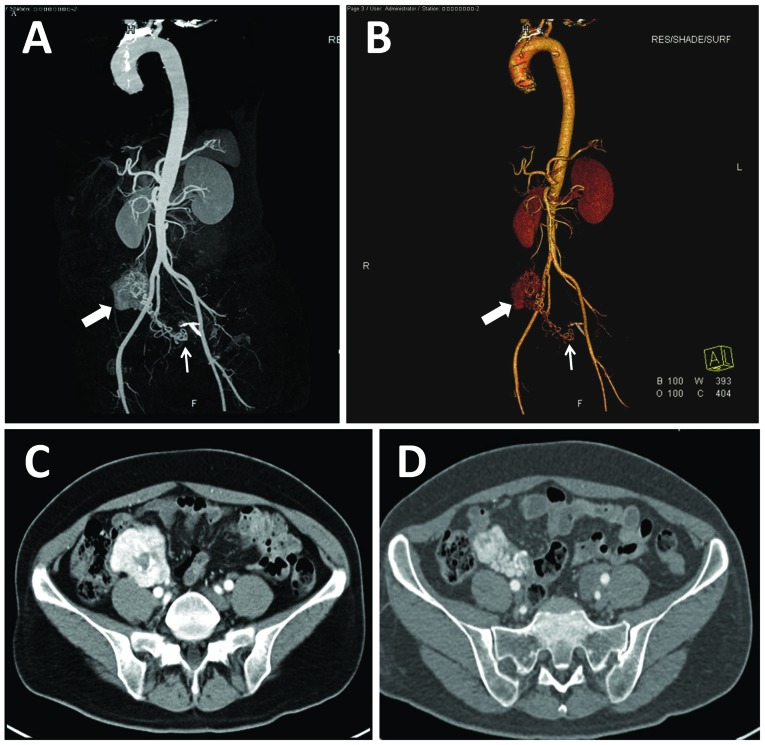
Computed tomography angiography (CTA) of an extra-adrenal pheochromocytoma. Abdominal aortic CTA post-processing: (A) maximum intensity projection and (B) shaded surface display show a highly vascularized tumor (thick arrow). The blood supply of the tumor is derived from the right ovarian and right internal iliac arteries (thin arrow). (C and D) Contrast axial CT demonstrates the tumor location at the superior surface of the right ovary, with clear borders.
